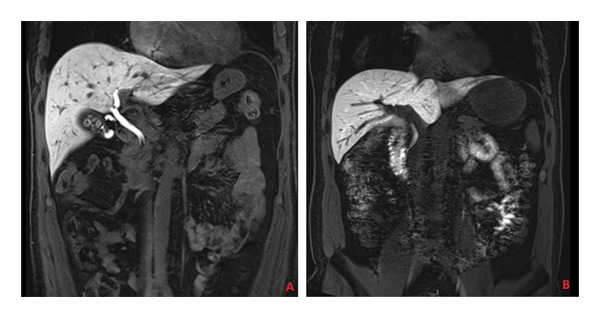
(A) In a 60‐year‐old male patient, fasting Gd‐EOB‐DTPA‐enhanced MRI hepatobiliary phase images reveal an obstructed duodenal papilla, preventing bile from entering the duodenum via the common bile duct. (B) A 50‐year‐old male patient with a patent duodenal papilla, shown on fasting Gd‐EOB‐DTPA‐enhanced MRI hepatobiliary phase images, demonstrates normal bile flow into the duodenum through the common bile duct.
